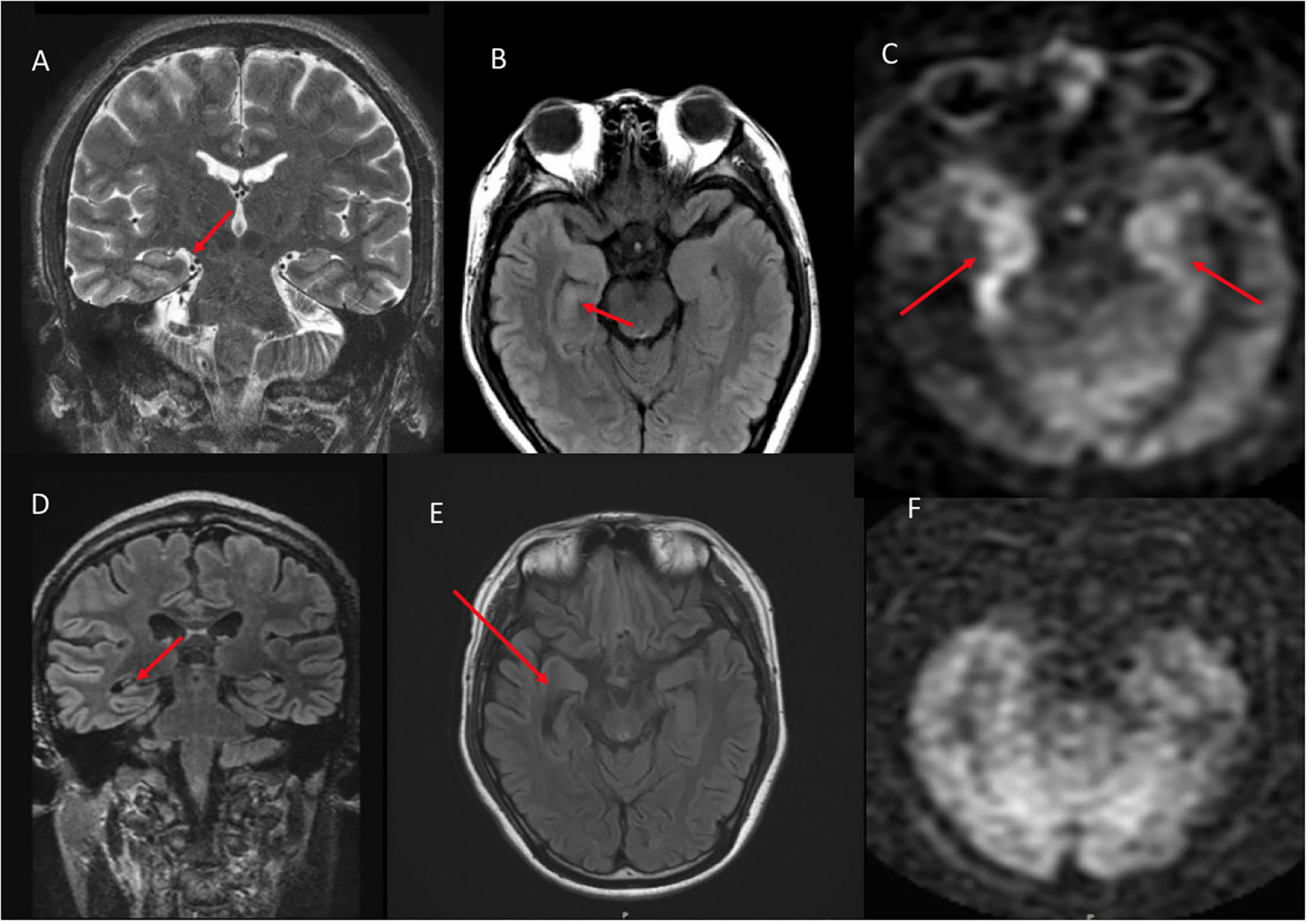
A 28 year old male who presented with seizures and altered mental status. (A) Coronal T2W shows mild right hippocampal edema. (B) Axial FLAIR shows subtle right hippocampal and amygadala hyperintense signal. (C) ASL demonstrates asymmetric right greater than left hyperperfusion. (D) 10 month follow up coronal FLAIR shows right hippocampal volume loss. (E) Axial FLAIR shows right hippocampal volume loss with associated ex vacuo dilatation of the right temporal horn. (F) ASL demonstrates resolution of the previous right greater than left hyperperfusion.
